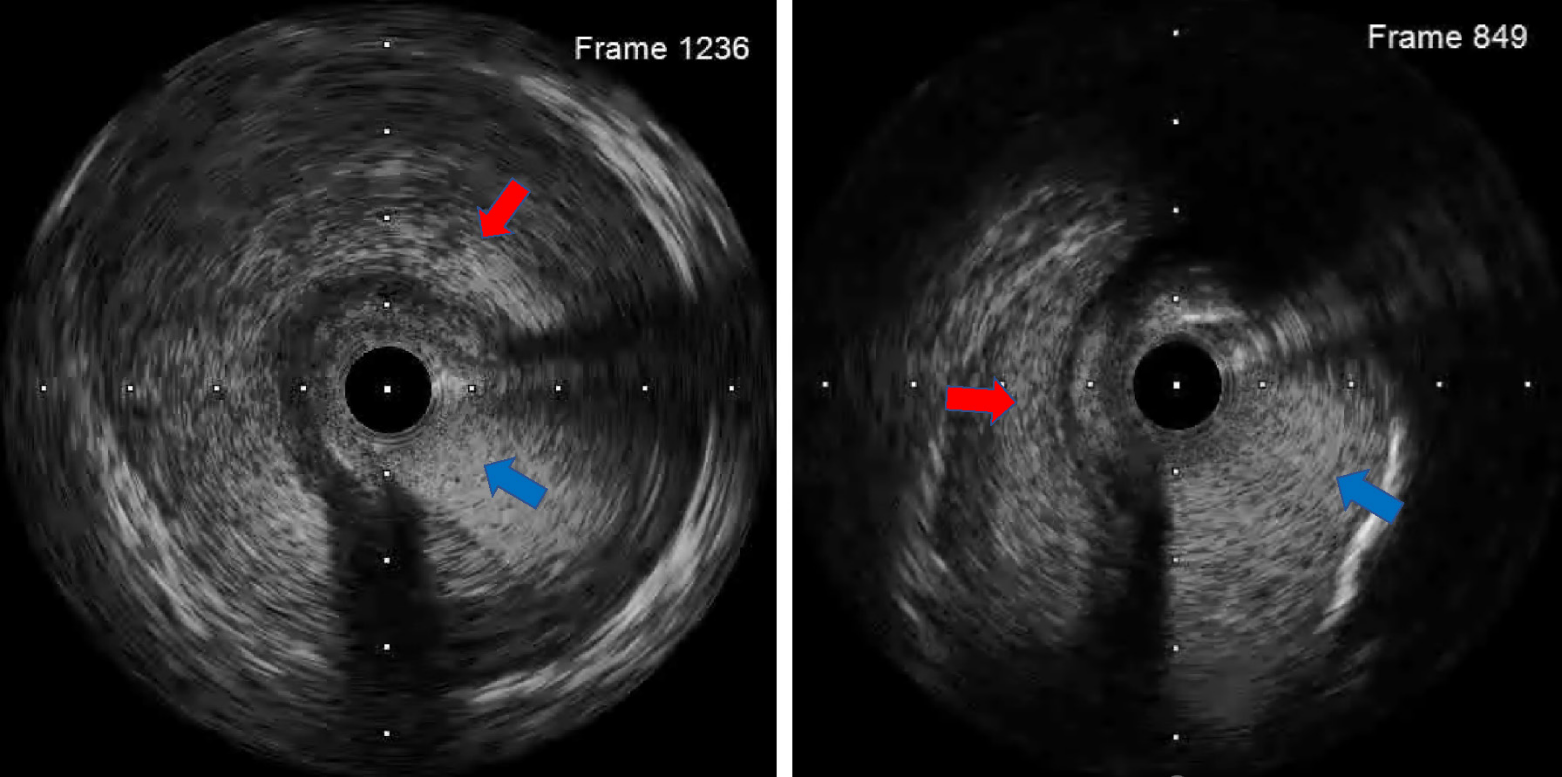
Intravascular ultrasound showed an extensive false lumen (*red arrow*) extending around the true lumen (*blue arrow*).
